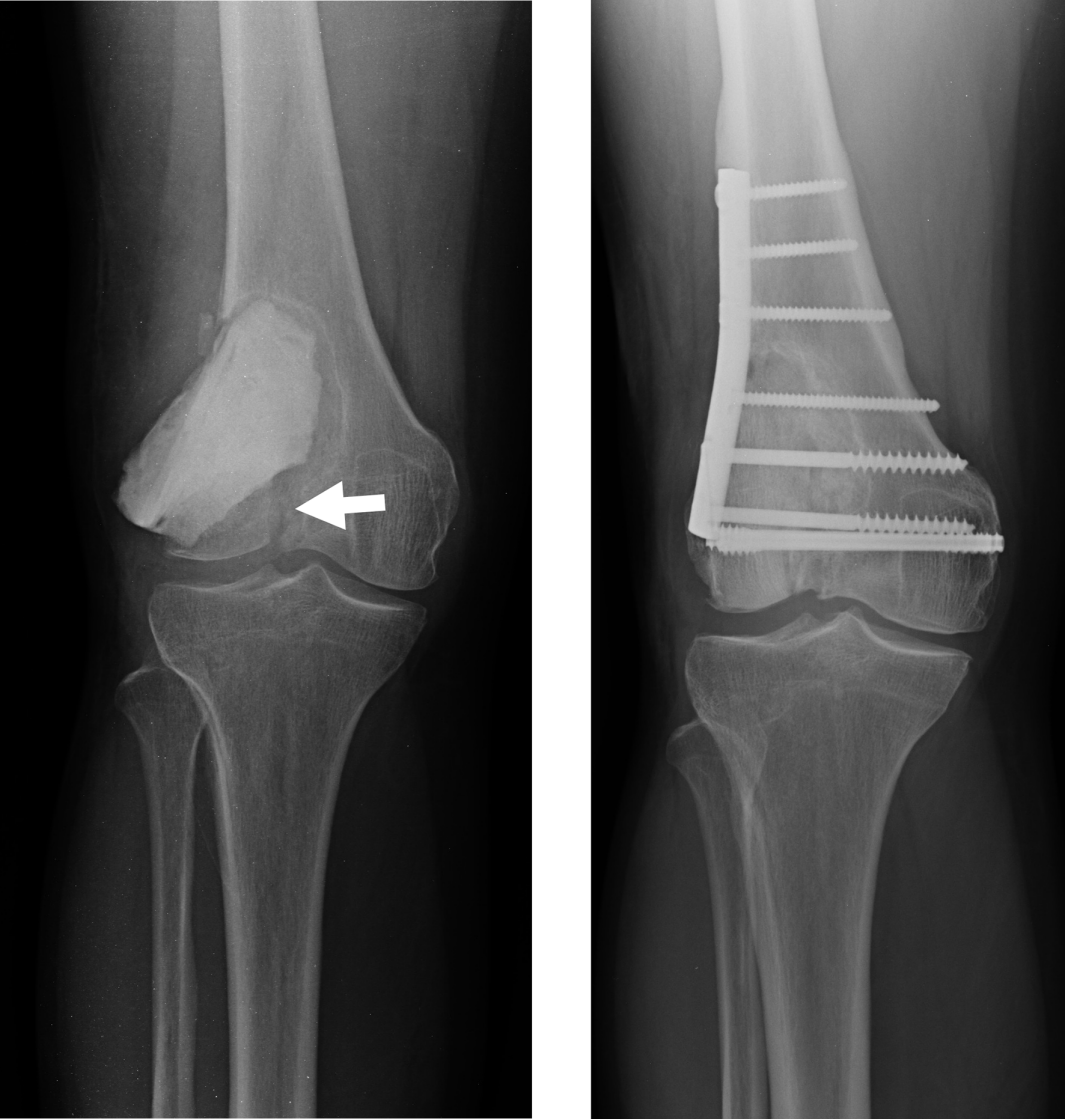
A, Radiography of a new fracture. The arrow indicates the fracture line. B, Radiography 2 years after removal of bone cement, filling of allografts, and osteosynthesis using a plate.
